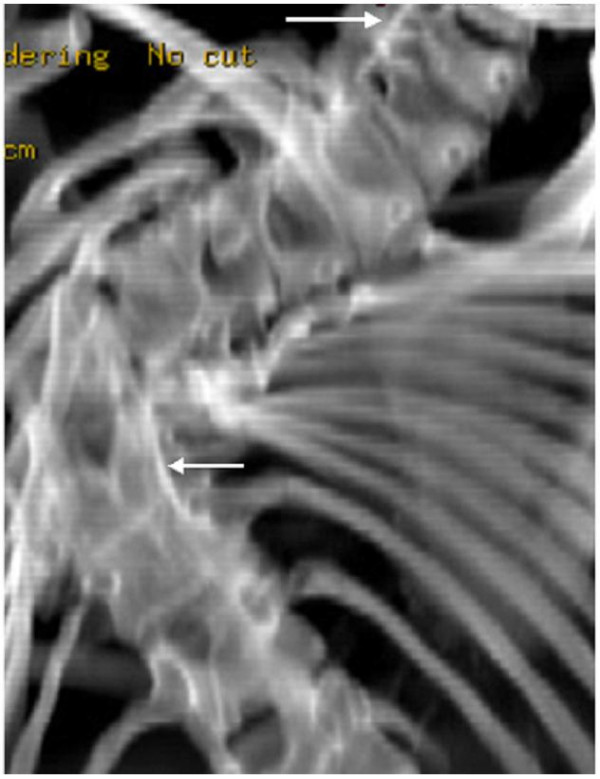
showed spinal segmentation defects and marked thoracic scoliosis (Cobb angle of 85 degree) with a unilateral unsegmented bar along the cervical and the thoracic spine, CT scan showed two unsegmented spinal bars-upper right arrow showed unsegmented unilateral cervical bar at the level of the C5-C6. Lower left arrow showed another unilateral unsegmented bar extending from T6-T10.
